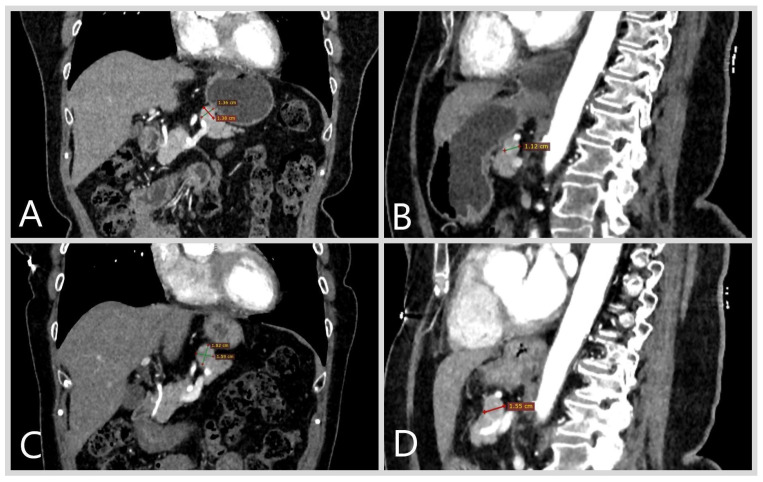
Multispiral computed tomography of the abdominal cavity, patient D. The neuroendocrine tumor of the pancreas body, with the indicated size, is highlighted with arrows. Frontal (A) and sagittal (B) views of the MSCT performed in February 2022. Frontal (C) and sagittal (D) of the MSCT performed in August 2023.
